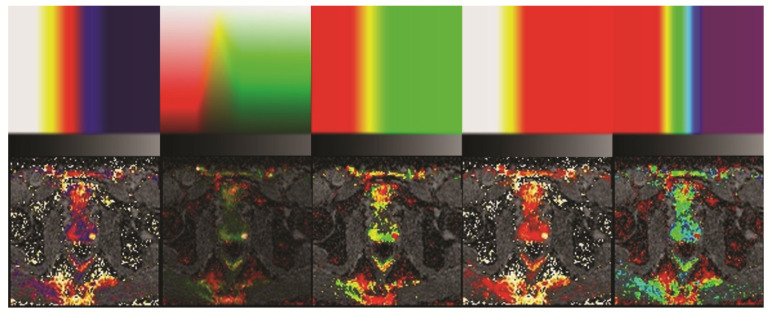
Example of different potential color maps. The map is shown in the top row and the corresponding image in the bottom row. The map is divided into two sections. The high DWI values are color-coded with different maps to better visualize the tumor (left posterior peripheral zone). The low DWI values represent the background (‘no-tumor zone’) and contain a vertical grayscale gradient. It can be noted that the highest ADC value is not white but 50% gray. This darkens the background.
